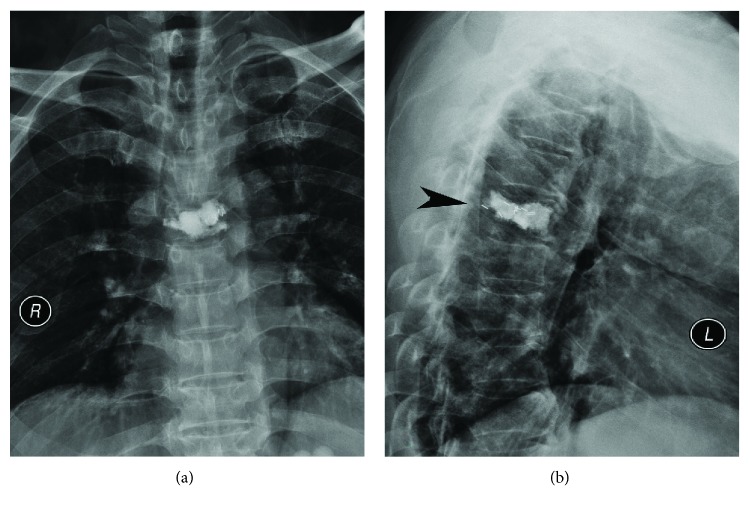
(a, b) The regular films of X-ray about the thoracic vertebrae show bone cement plus ^125^I seeds in the T4 body. Black arrow indicates the location of ^125^I seeds close to the back wall of the vertebra.
